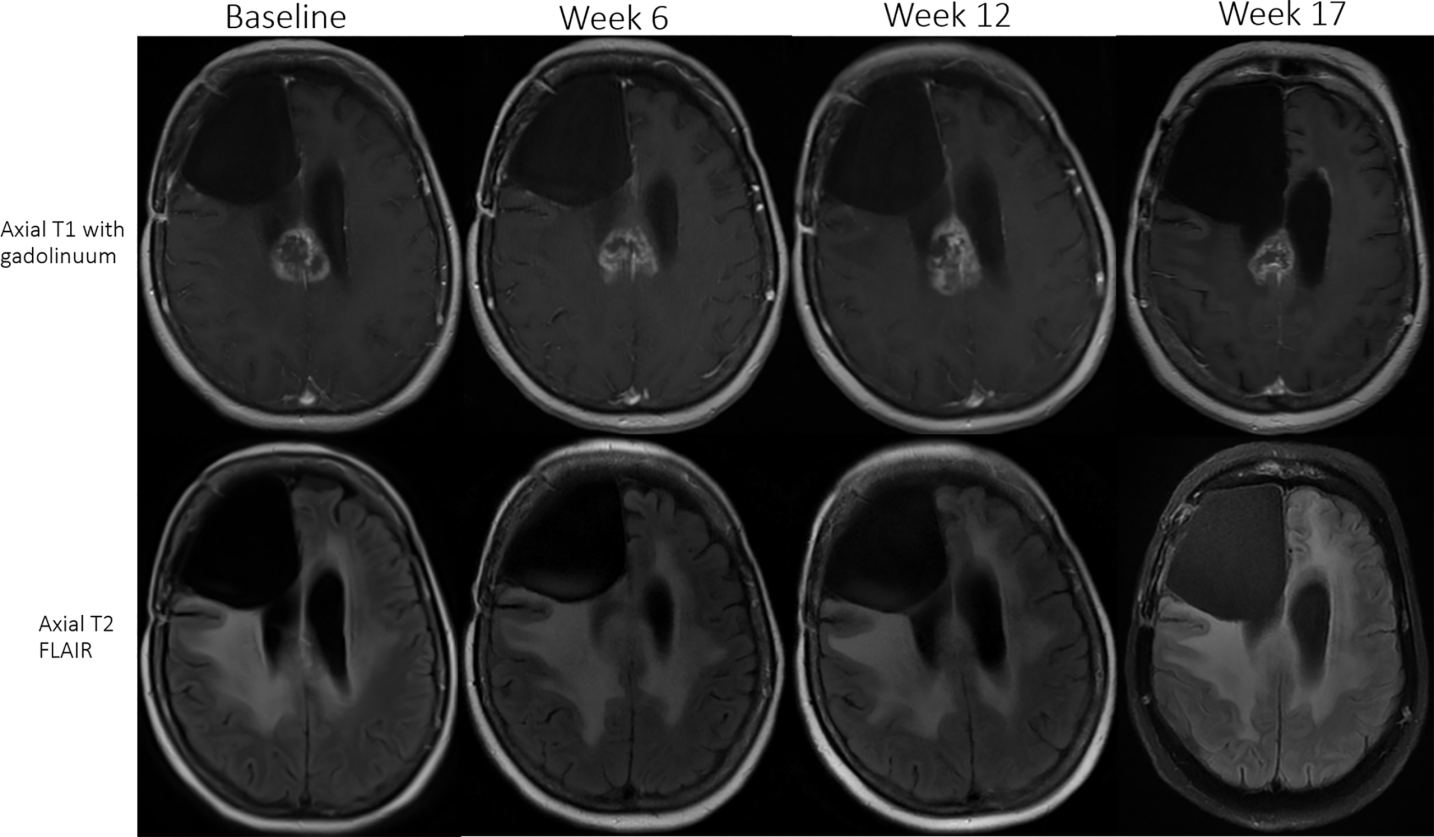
Patient 11 (received nivolumab and ipilimumab) had an initial 27% increase in enhancing lesion burden at first MRI (week 6), 13% decrease at the next follow-up MRI (week 12), and subsequently a 36% decrease (week 17) in size of the enhancing lesion. The patient remained stable on ICB for two additional months until treatment was discontinued due to declining performance status in the setting of a stable MRI scan.
